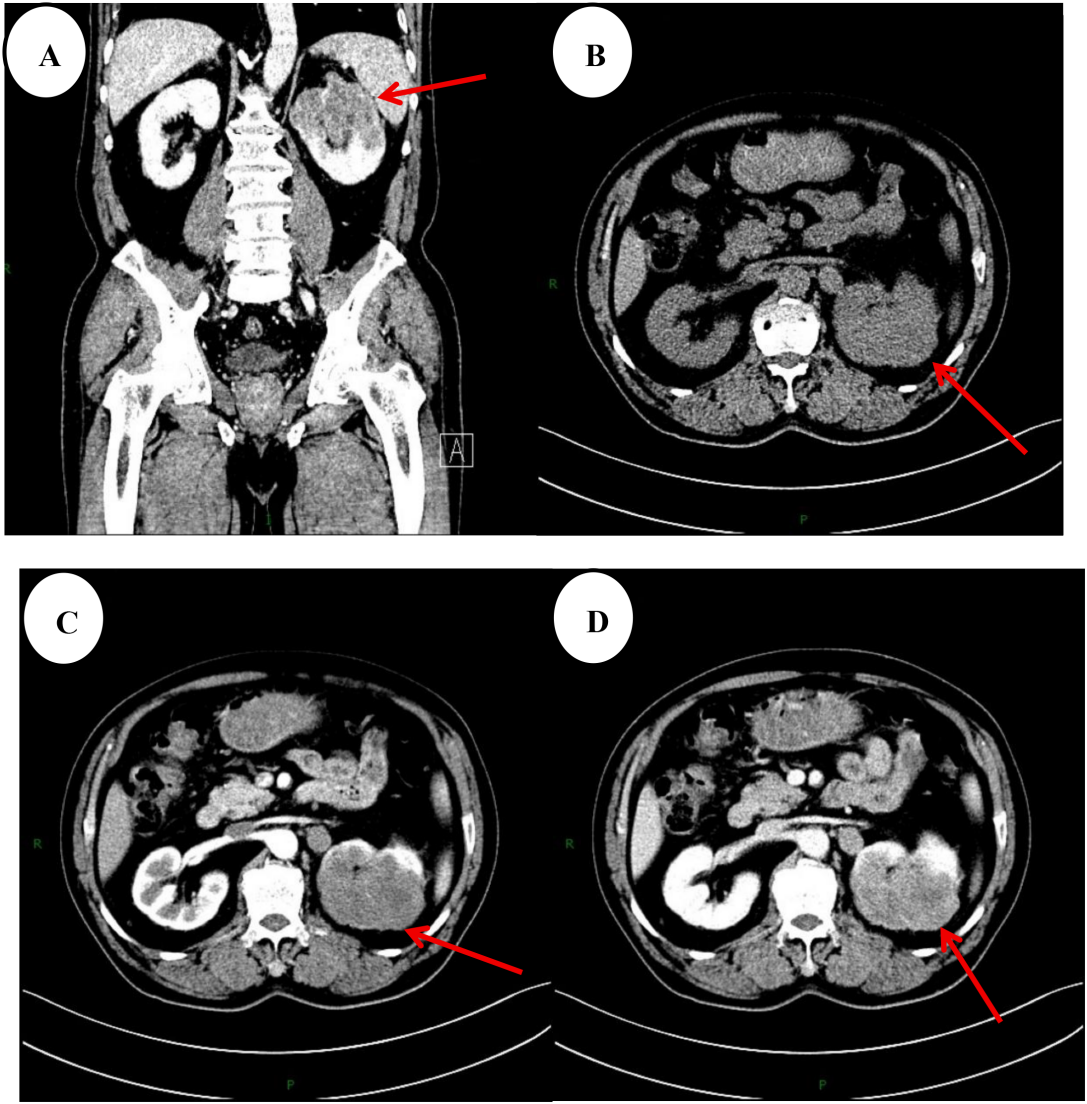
(A–D) Unenhanced CT images demonstrate a soft-tissue mass at the upper pole of the left kidney with ill-defined margins and heterogeneous attenuation. Contrast-enhanced imaging reveals mild, heterogeneous enhancement within the mass.
